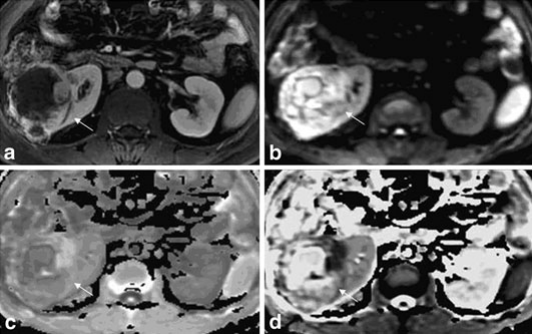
Right renal clear cell carcinoma, grade II with in a patient of 63 years old (male). a Enhanced scan in medulla phase, the substantial part of the right kidney lesions showed irregular peripheral enhancement, and the central part with necrosis and cystic degeneration showed no enhancement. b DWI lesions showed mixed high signal intensity in both the tumor parenchyma and central cystic area. c The EADC lesions showed peripheral high signal and central low signal, which was in accordance with what was displayed by enhanced scanning. In the background of gray kidneys, the lesion was highlighted with a clear internal structure. The high signal indicates the substantial part of the tumor. d ADC lesions showed mixed high signal, with high signal in central necrosis part and low signal in surrounding parenchyma part. Compared with the enhanced scan and EADC map, the peripheral part of the kidney was less legible by ADC map. The abdominal intestinal signal was cluttered. Compared with the ADC map, EADC map showed much clean background and much clear image
